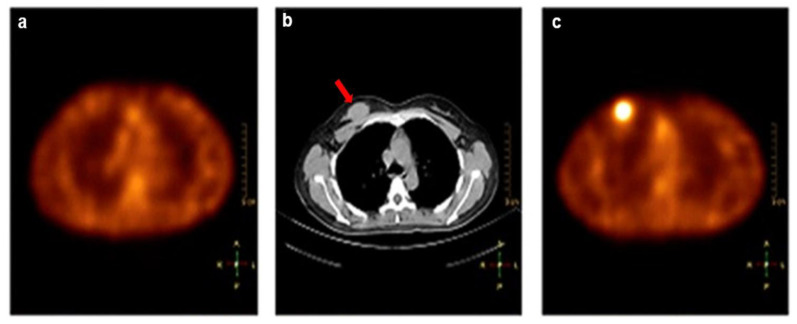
SPECT/CT images of a breast tumor that is positive for GRPR but negative for integrin αvβ3 expression. (a) [^99m^Tc]Tc-3P4-RGD2 SPECT had no tracer uptake in the lesion. (b) CT scan showed a mass in the right breast (arrow). (c) [^99m^Tc]Tc-RGD-BBN SPECT demonstrated high uptake in the lesion. (Reprinted from Ref. [31]).
